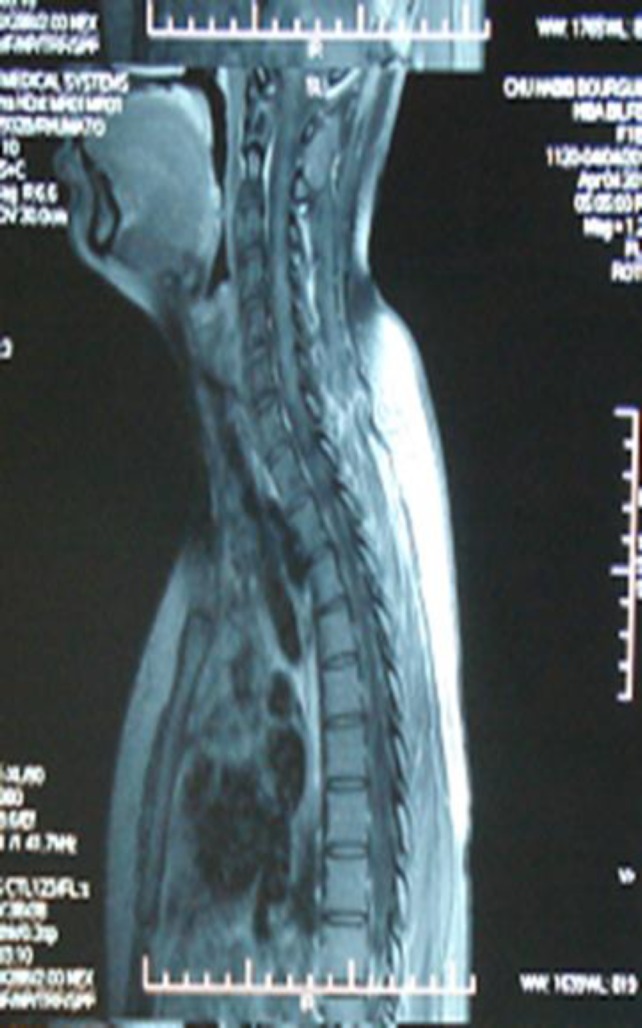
The spinal cord MRI revealed T2-weighted images a homogeneous hyperintensity was found extending along the 1^st^ and 2^nd^ cervical vertebrae and demonstrated moderate gadolinium enhancement
